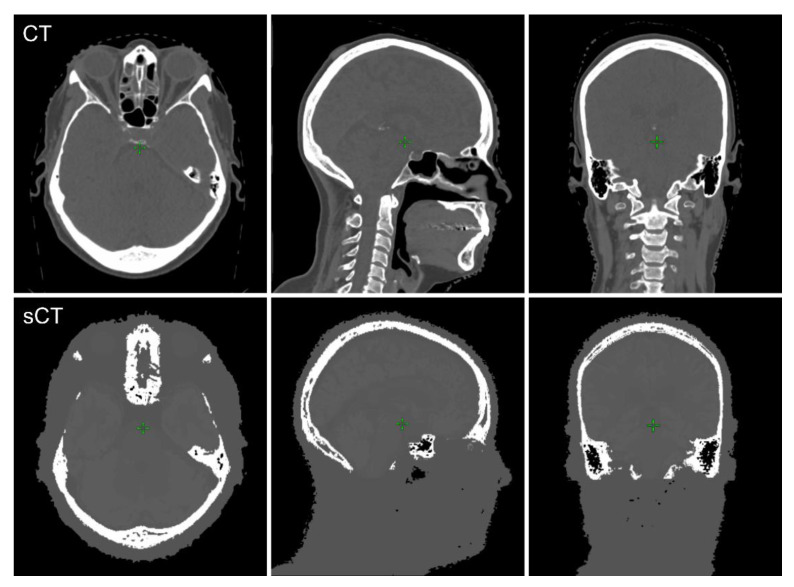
Case example of the CT (top) and generated sCT (bottom) image pairs in transversal, sagittal, and coronal directions. (CT: computed tomography, sCT: synthetic computed tomography).
